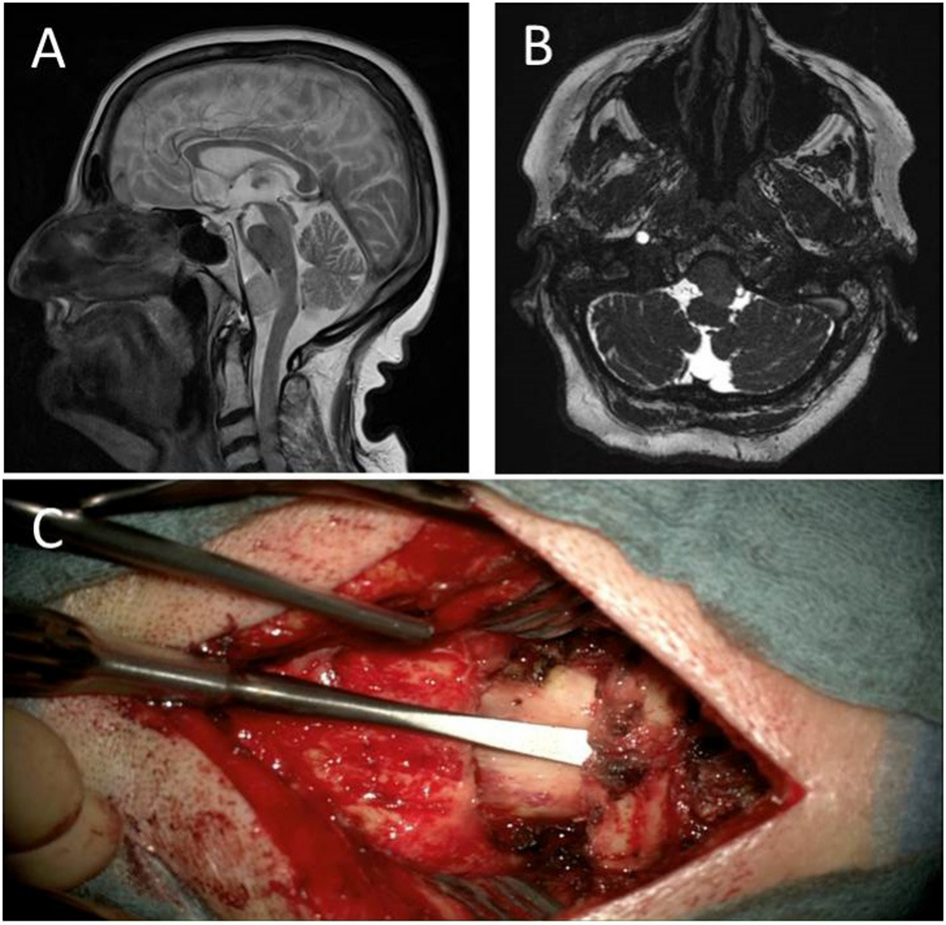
Preoperative sagittal T2-weighted (A) MRI of case 3 depicting a foramen magnum and lower clivus meningioma with a cranial extension up to the vertebral junction. Axial 3D-CISS images (B) show a sufficiently wide craniocervical cerebrospinal fluid (CSF) space and a bilaterally well distinguishable vertebral artery. After preparation of the paravertebral muscles, an Adson retractor was placed exposing all boney structures from occiput down to C2 (C) showing a wide enough space for tumor resection.
